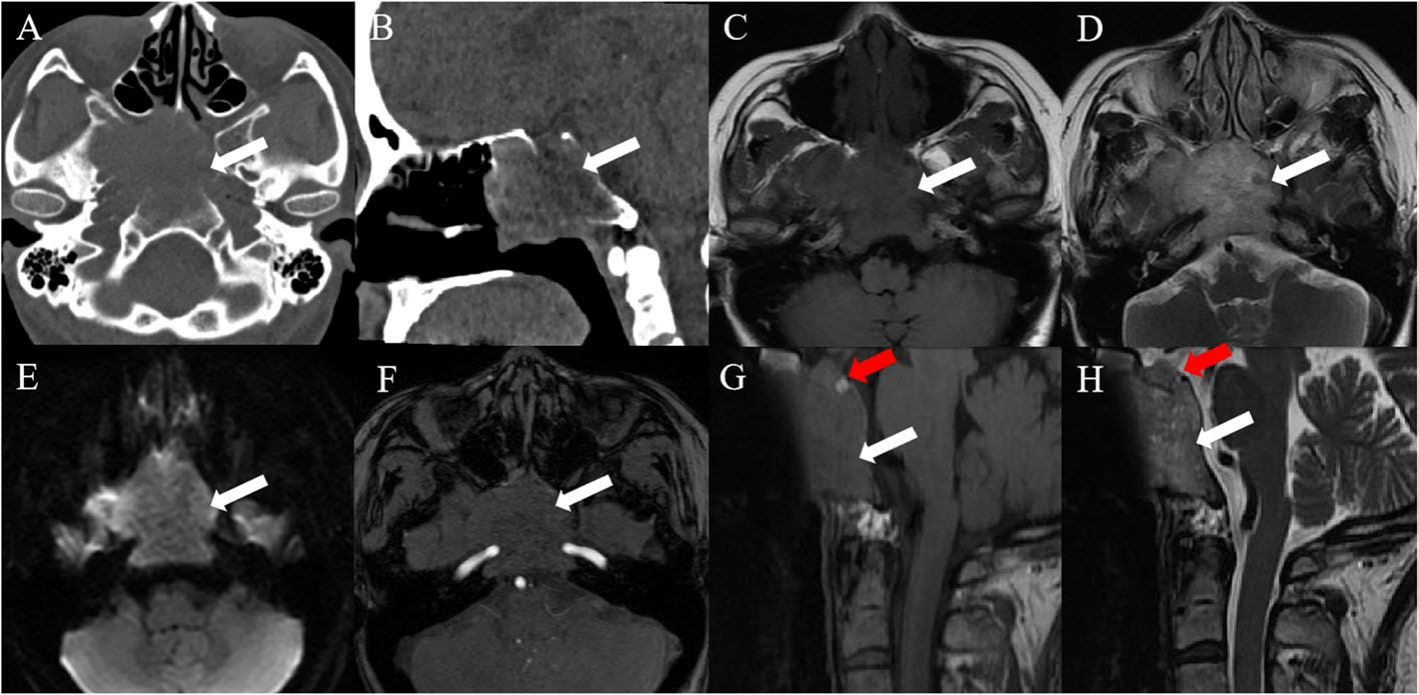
Preoperative CT and MRI. A Axial CT image without contrast demonstrates an ill-defined mass (white arrow) in the sphenoid sinus and clivus eroding the adjacent bone. B Sagittal CT image displays the relationship between the mass (white arrow) and adjacent structures. C-F The mass (white arrow) presents as low to high signal on T1-weighted and T2-weighted images with slightly diffusion restriction and vessels involvement. G-H The mass (white arrow) was outside the sellar without a direct connection to the normal pituitary tissue (red arrow)
